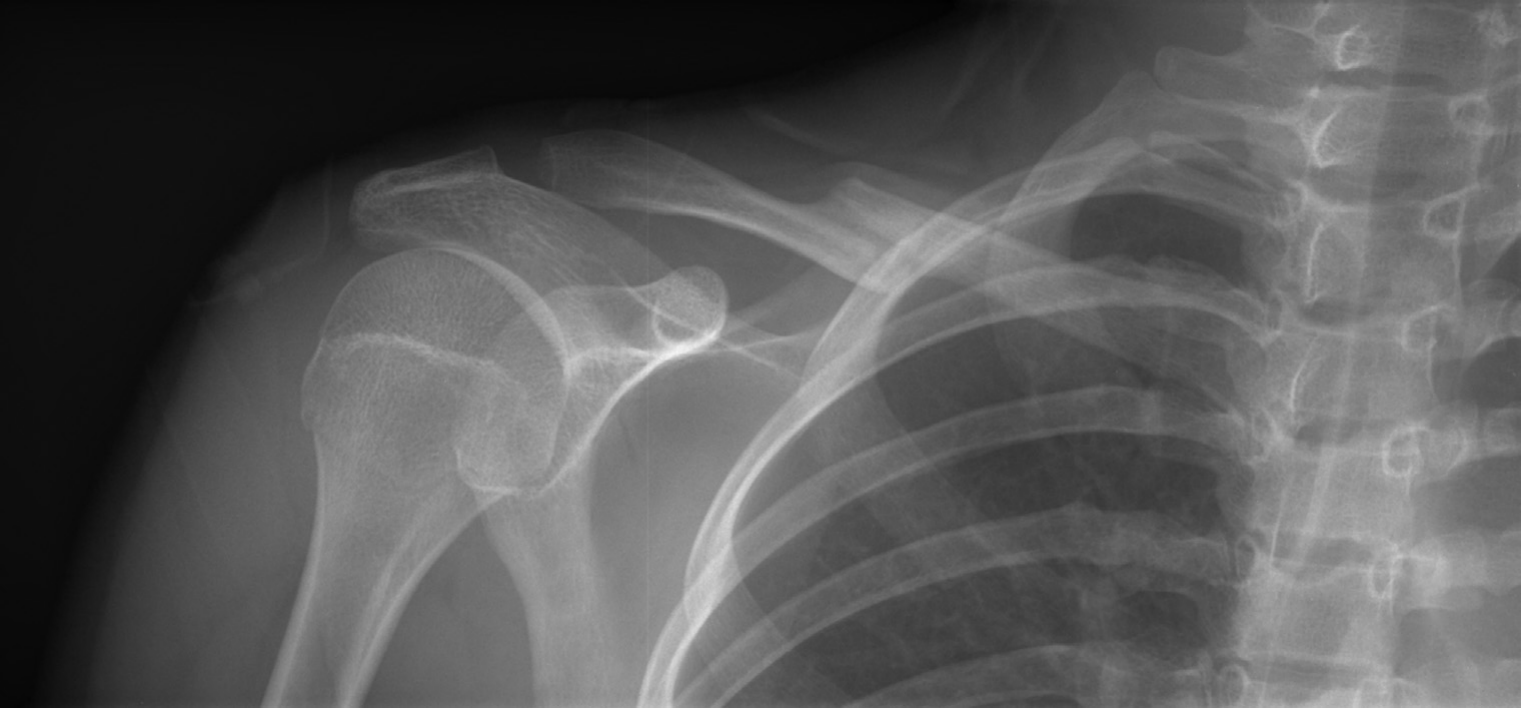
Anterior-posterior radiograph of right clavicle at last follow-up approximately 5 months following initial injury.
